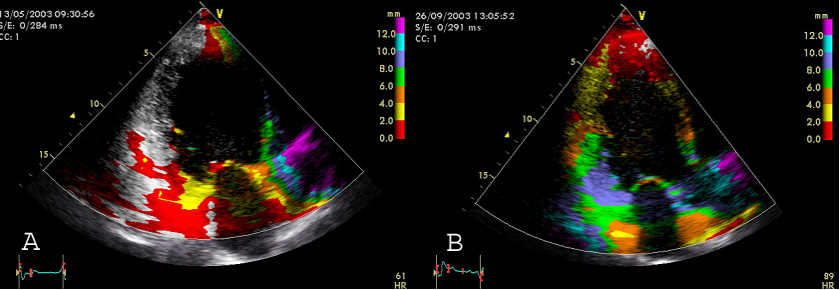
Successful BVP documented by Tissue Tracking in apical two chamber view. A) Before BVP, there is a dilated ventricle with reduced systolic displacement (max displacement = 8 mm) in the septum and DLC in the inferior wall (no colour-coding) B) After three months of BVP, there is a reduction of left ventricular dilatation (reverse remodelling), increased tracking values, a basal-apical gradient and no DLC regions anymore.
